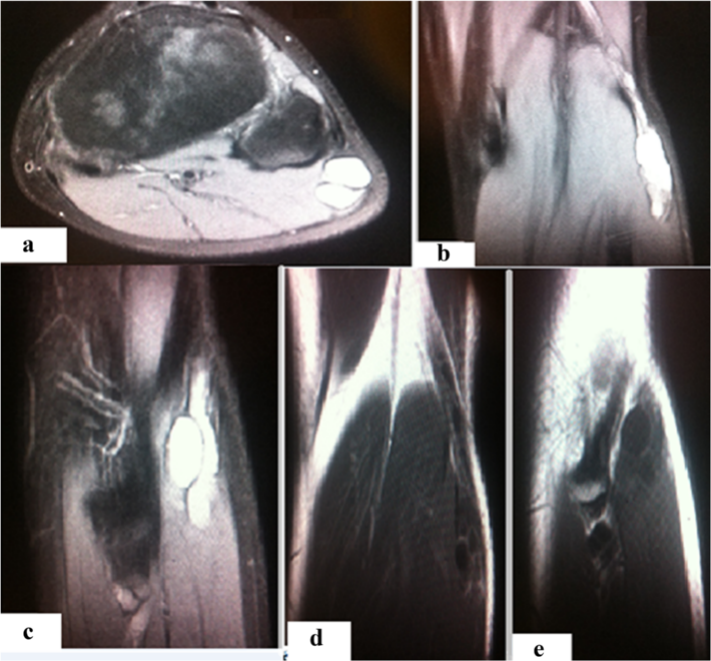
Magnetic resonance imaging. a Proton density fat-saturated cross-section showing a lesion projecting on to the upper outer quadrant of the patient’s popliteal fossa, a rounded area of high signal intensity and a few thin septa. b Proton density fat-saturated coronal section showing a lesion projecting on to the upper outer quadrant of the popliteal fossa, a heterogeneous area of high signal intensity and a few thin septa. c Proton density fat-saturated sagittal section showing a lesion projecting on to the upper outer quadrant of the popliteal fossa, a heterogeneous oblong area of high signal intensity and a few thin septa. d Coronal T1 sequence after gadolinium injection showing peripheral contrast enhancement and septa at the upper outer quadrant of the popliteal fossa. e Sagittal T1 sequence after gadolinium injection showing peripheral contrast enhancement and septa at the upper outer quadrant of the popliteal fossa
